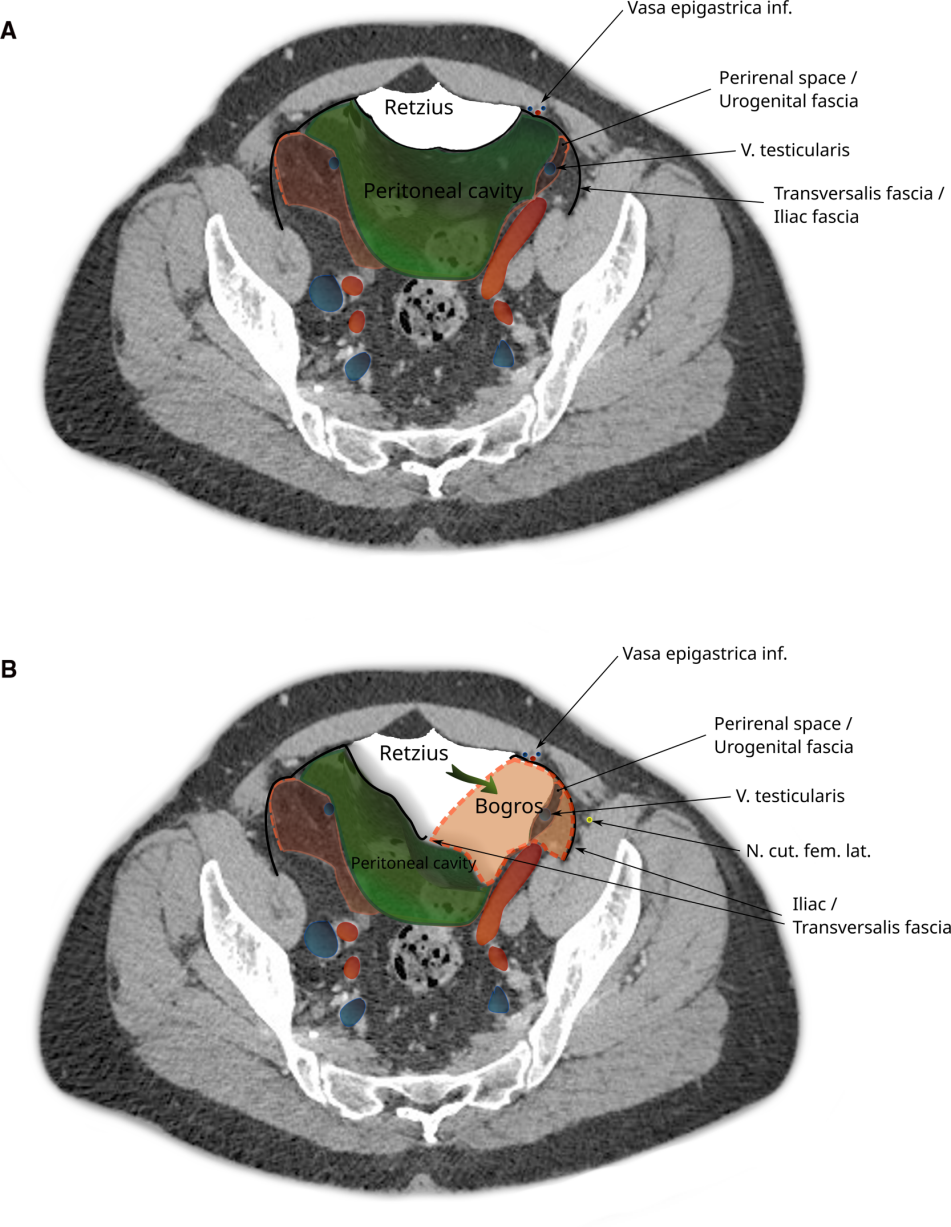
CT image of the pelvis showing dissection during a total extraperitoneal hernioplasty (TEP). (A) The peritoneal sac is displaced and the space of Retzius dissected. (B) The space of Bogros is opened by cleaving the transversalis fascia. Note that the space of Bogros contains the spermatic vessels and tissues from the perirenal space enveloped by the urogenital fascia that extends from the abdomen into the pelvis.
